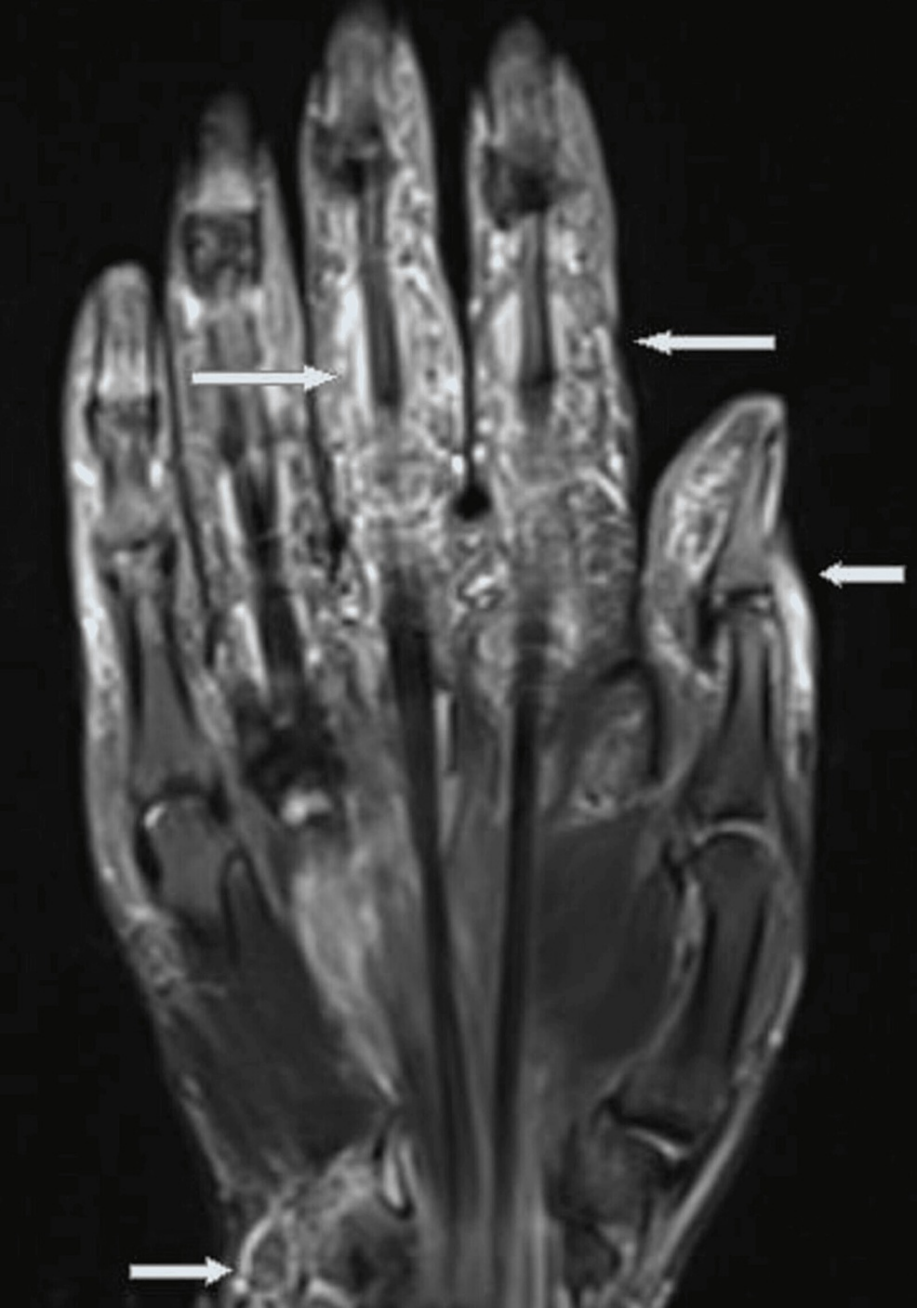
Tenosynovitis of the flexor tendon sheaths, synovitis of the intercarpal, and 2-3 metacarpophalangeal joints and medullary bone marrow edema of the capitatum were detected in the magnetic resonance imaging of the hand.
